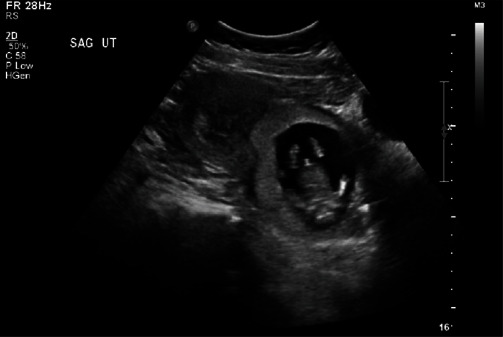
Transabdominal and sagittal ultrasound view demonstrating cervical pregnancy with empty endometrial cavity.
